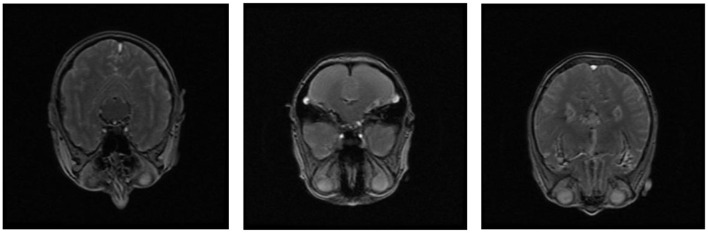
Sample brain MRI of CN, MCI, and AD.
